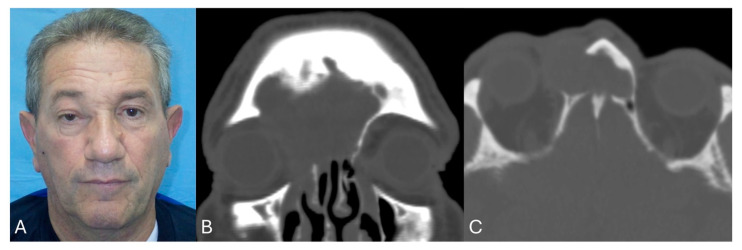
Case example pre-surgery. (A) Clinical photo with evidence of dystopia and globe dislocation; (B) pre-operative CT coronal view and (C) pre-operative CT axial view, with evidence of frontal and orbital involvement by mucocele.
